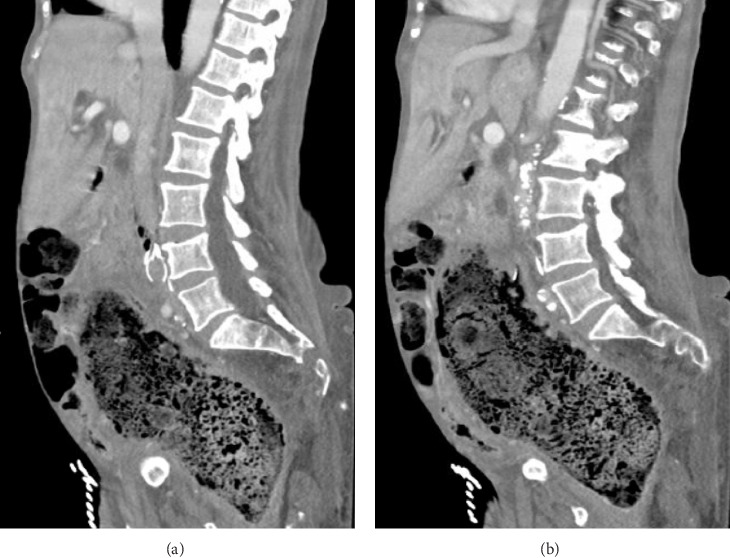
CT abdomen and pelvis sagittal views. Intracaval air cranial to filter arms (a). Filter leg in colon wall (b).
